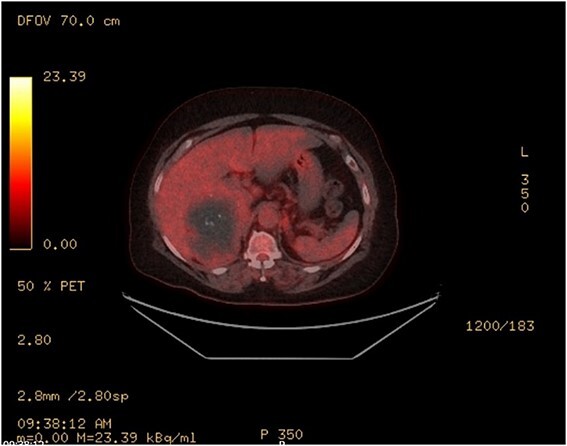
PET—large heterogenous complex right adrenal mass that measured up to 10 cm is largely photopenic with a mild peripheral rim of FDG accumulation (SUV max 3.4) at a level similar to adjacent physiologic hepatic activity.
